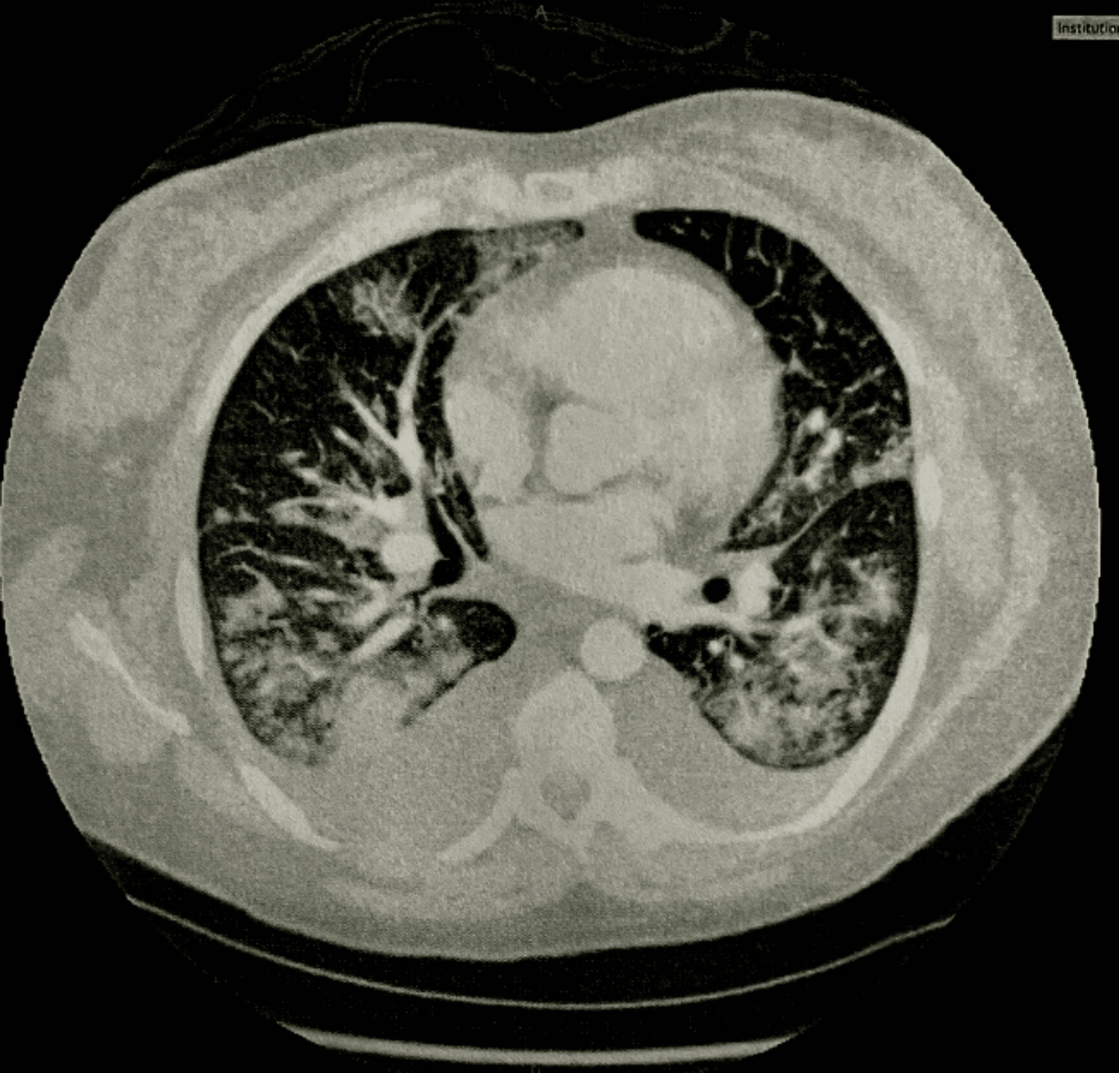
Computed tomography angiography (CTA) chest performed post-operative day one Impression reported pulmonary edema with bilateral pleural effusions, extensive groundglass and airspace disease, a developing consolidation in the right lower lobe, as well as a prominent central pulmonary artery.​
